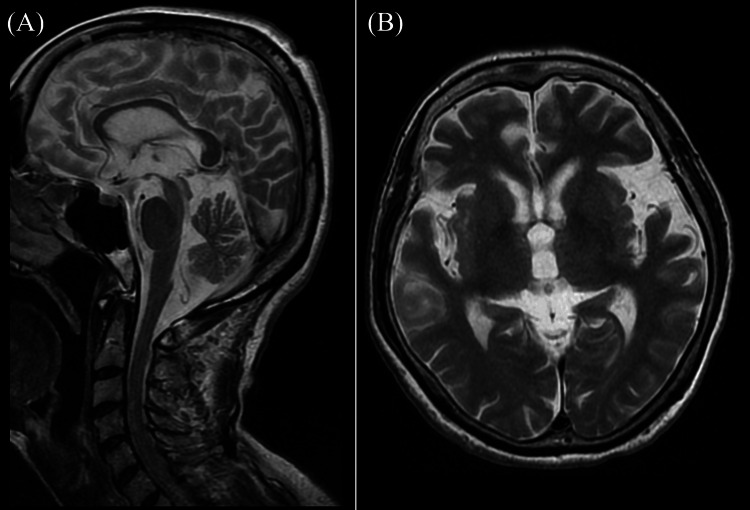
Brain magnetic resonance imaging (MRI) findings. (A, B) Brain MRI revealed no significant abnormalities, including brainstem atrophy or basal ganglia region abnormalities.
